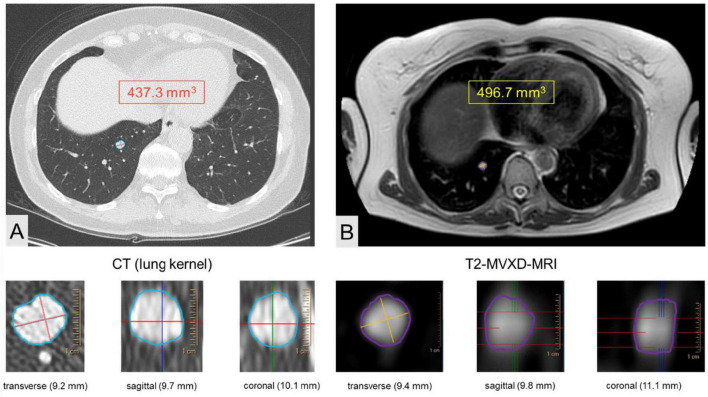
Volumetric assessment of a lung nodule in the right lower lobe using CT (A) and T2 weighted MVXD MRI (B) in a 56-year-old study patient.
